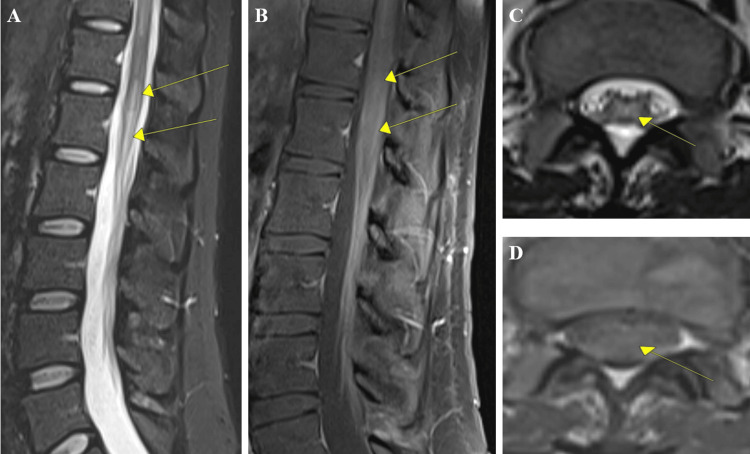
MRI of the lumbar spine with and without contrast. A-B) MRI of lumbar spine abnormal T2 hyperintensities in the spinal cord and distal conus medullaris with mild pathological contrast enhancement of the conus medullaris and the cauda equina. C-D) Axial cuts with T2 hyperintensity of the conus medullaris with mild contrast enchantment.
